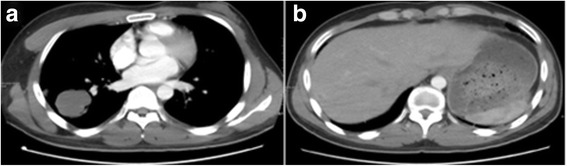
a (left): CT showing the initial reduction in size of multiple lesions after BEP chemotherapy. The largest nodule is 4.7 × 4.2 cm. b (right): The enhanced CT shows masses of heterogeneous density within the liver parenchyma
